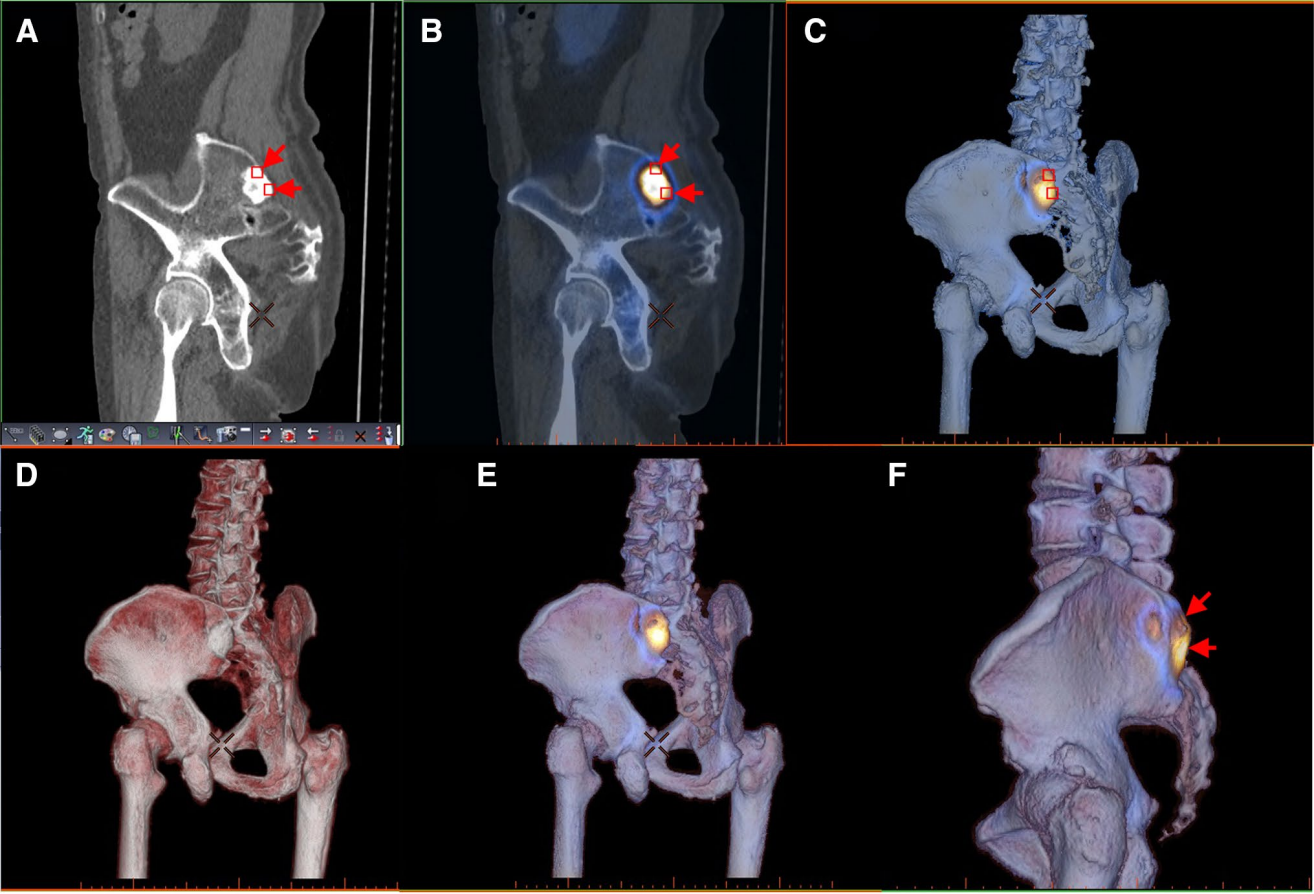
Data expression of bone SPECT into 3D volume-rendered CT images in the patient, as shown in Fig. 1. In the conventional CT, the bone metastasis shows a high density area according to CT values (a, *arrows*). In two-dimensional SPECT/CT fusion image, the lesion is colored according to SPECT values at the corresponding site (b, *arrows*). In our data expression of bone SPECT into 3D volume-rendered CT images (c), three-dimensional bone structures are colored according to SPECT values at the surface of the visualized bones (a–c, *small squares*). To evaluate CT-based bone structure and bone metabolism, volume-rendered CT image (d) is blended with Image C (e). No extraosseous uptake is seen with our method (f, *arrows*)
